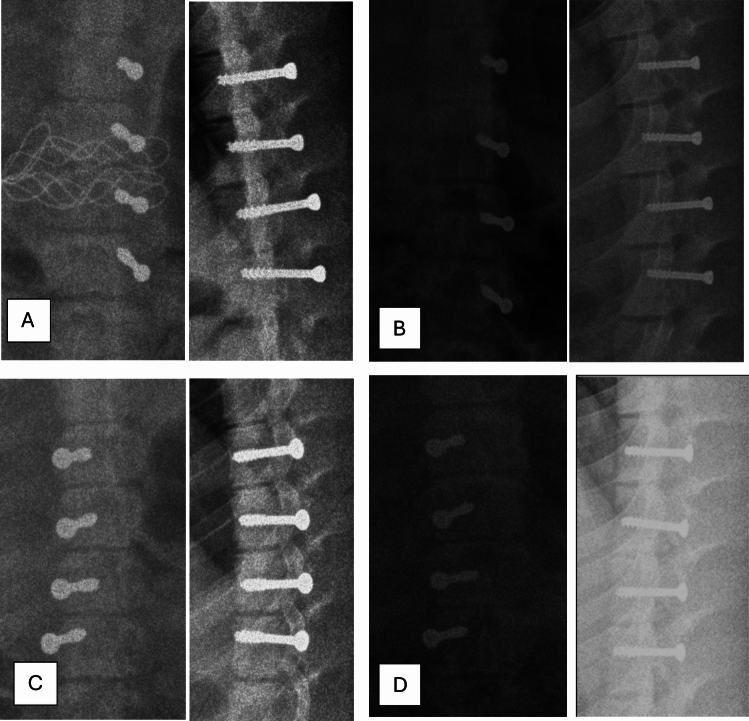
A-D Anteroposterior and lateral radiographs demonstrating pedicle screw instrumentation at levels T9 to T12. Case 1:A immediately postoperatively andB at 3-month follow-up. Case 2:C immediately postoperatively andD at 3-month follow-up
